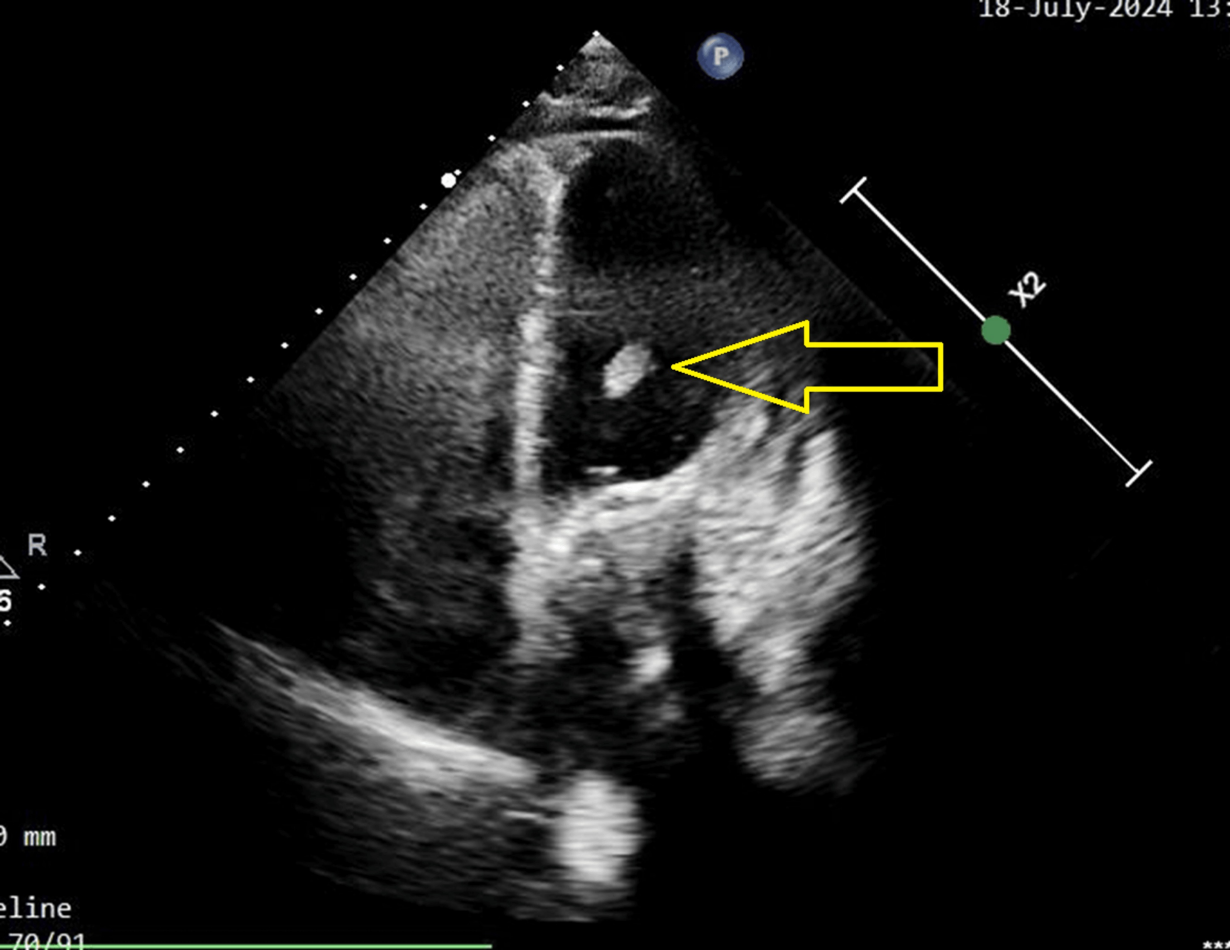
Postprocedure 2D echocardiography image showing MVR (yellow arrow) 2D: two dimensional; MVR: mitral valve replacement
